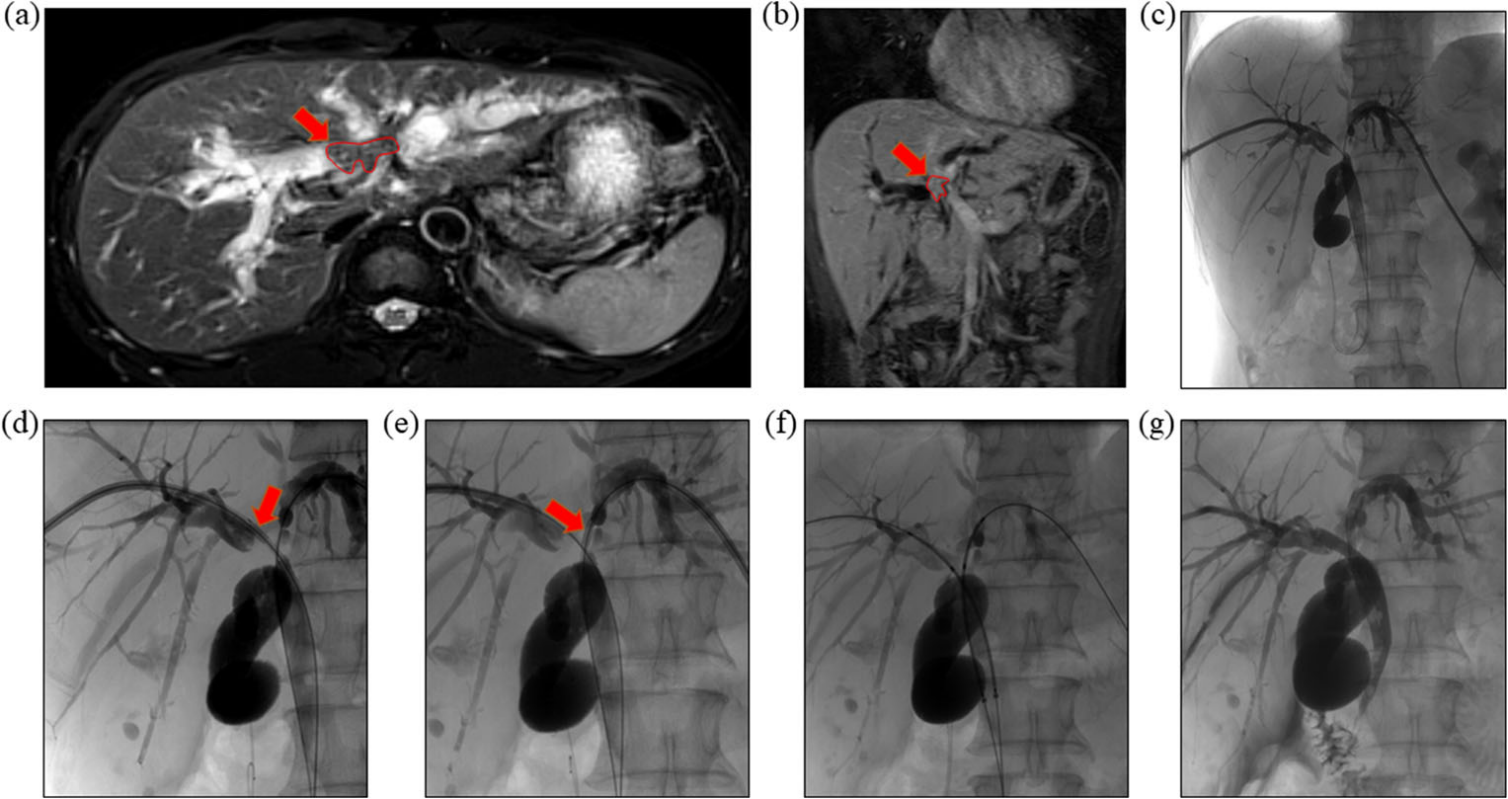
Intra Intraluminal radiofrequency ablation and double stent-by-stent deployment. a, b MRCP and contrast-enhanced CT showed perihilar cholangiocarcinoma presented malignant obstructive jaundice. c–e By adding a hard-exchange guide wire and sending into the ablation catheter, radiofrequency ablation (red arrow) was performed on the left and right bile duct stenosis segment. f, g After balloon expansion, the uncovered metal stent was implanted, and angiography showed that the stent was expanded well, and the common bile duct was restored unobstructed
